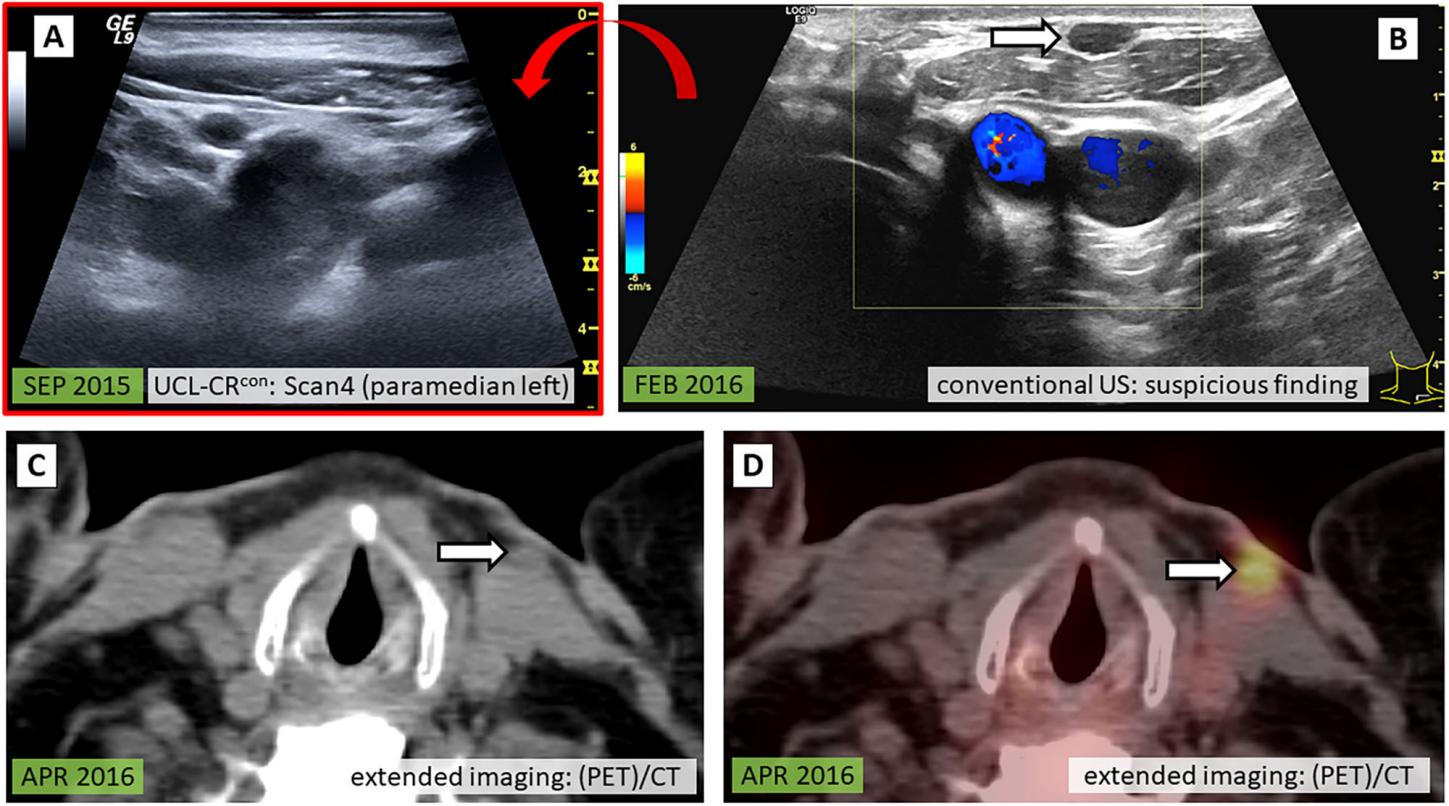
Patient case I: UCL-CR^con^ with confirmed diagnosis. 54-year-old male at initial diagnosis 2008 (FTC pT3 cN1 cM0). Conventional US revealed a suspicious subcutaneous nodule measuring 5 mm [B, white arrow] along with increasing TG levels (SEP 2015: 14.8 ng/ml, FEB 2016: 35.1 ng/mL, unstimulated). The retrospective PACS review of the UCL from SEP 2015 [A] confirmed that the identified lesion is new and thus highly suspicious for a cervical FTC relapse. Consequently, additional extended imaging was conducted. The nodule was reproduced on CT [C, white arrow] and showed elevated FDG uptake on PET/CT [D, white arrow]. The subsequent surgical removal confirmed a late relapse of FTC. UCL-CR^con^ standardized cervical ultrasound cine loops with clinical relevance - confirmation of a suspicious finding seen on conventional live ultrasound, US ultrasound, PET/CT positron emission tomography computed tomography, FTC follicular thyroid carcinoma, TG thyroglobulin
